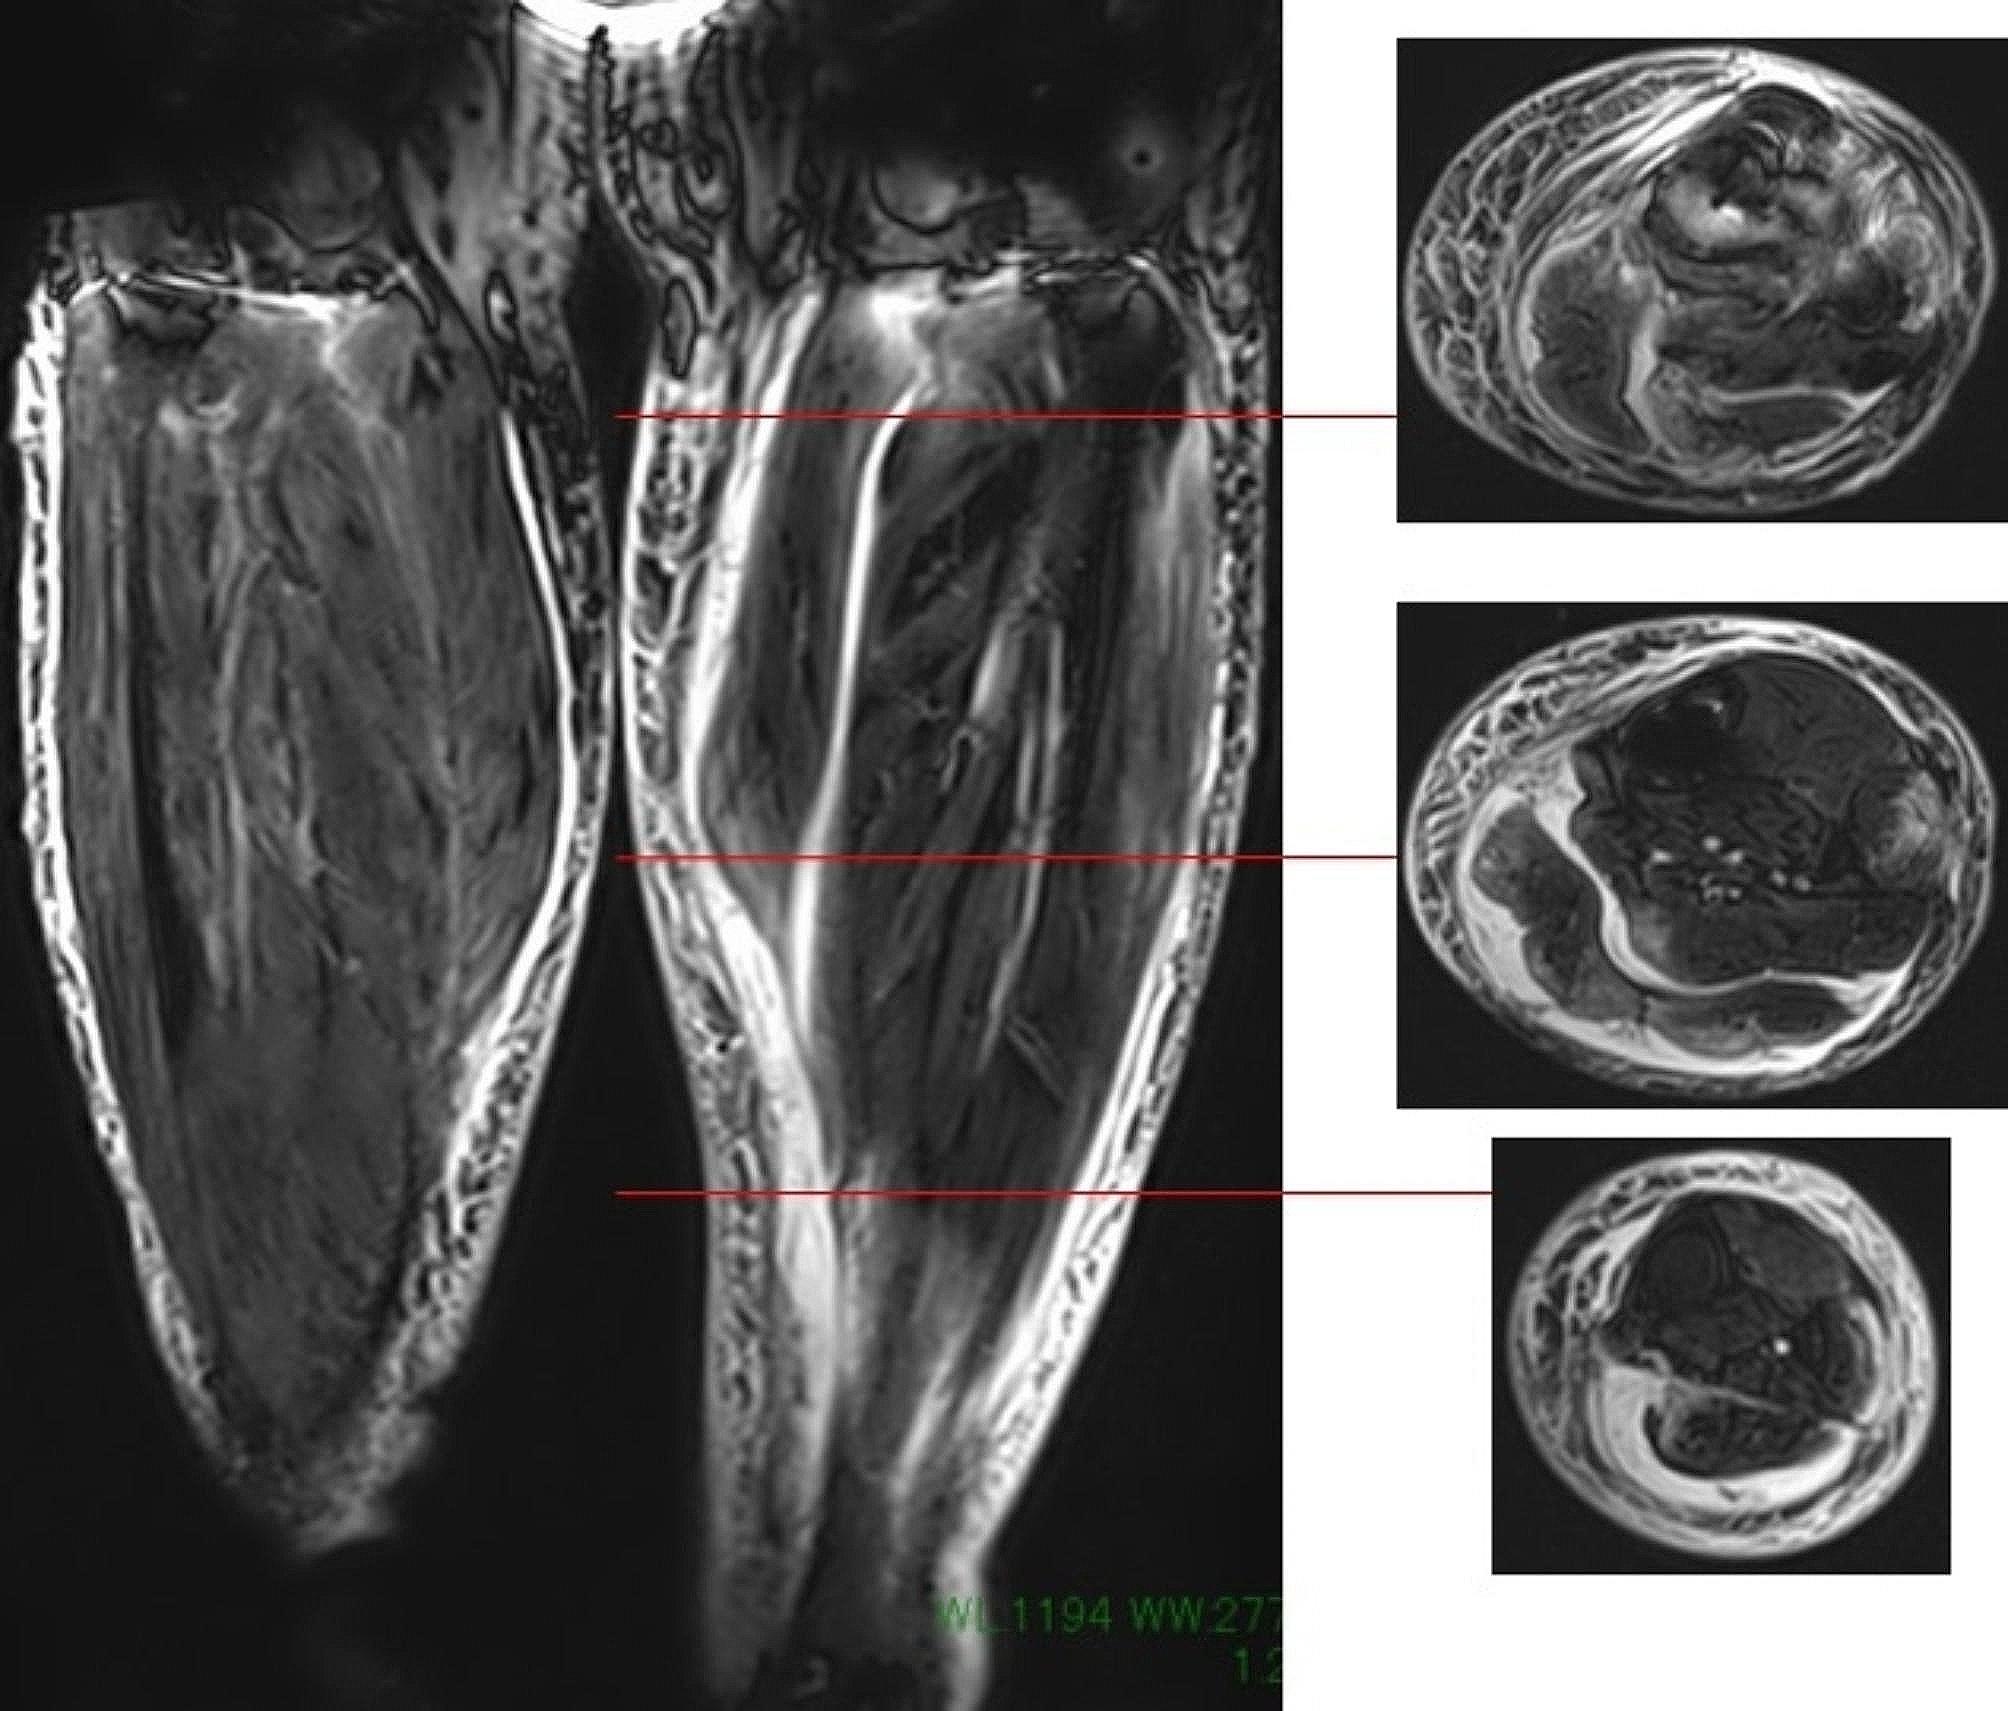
Suspicion of necrotizing soft tissue infection due to fluid accumulation around the gastrocnemius muscle on the leg MRI image
